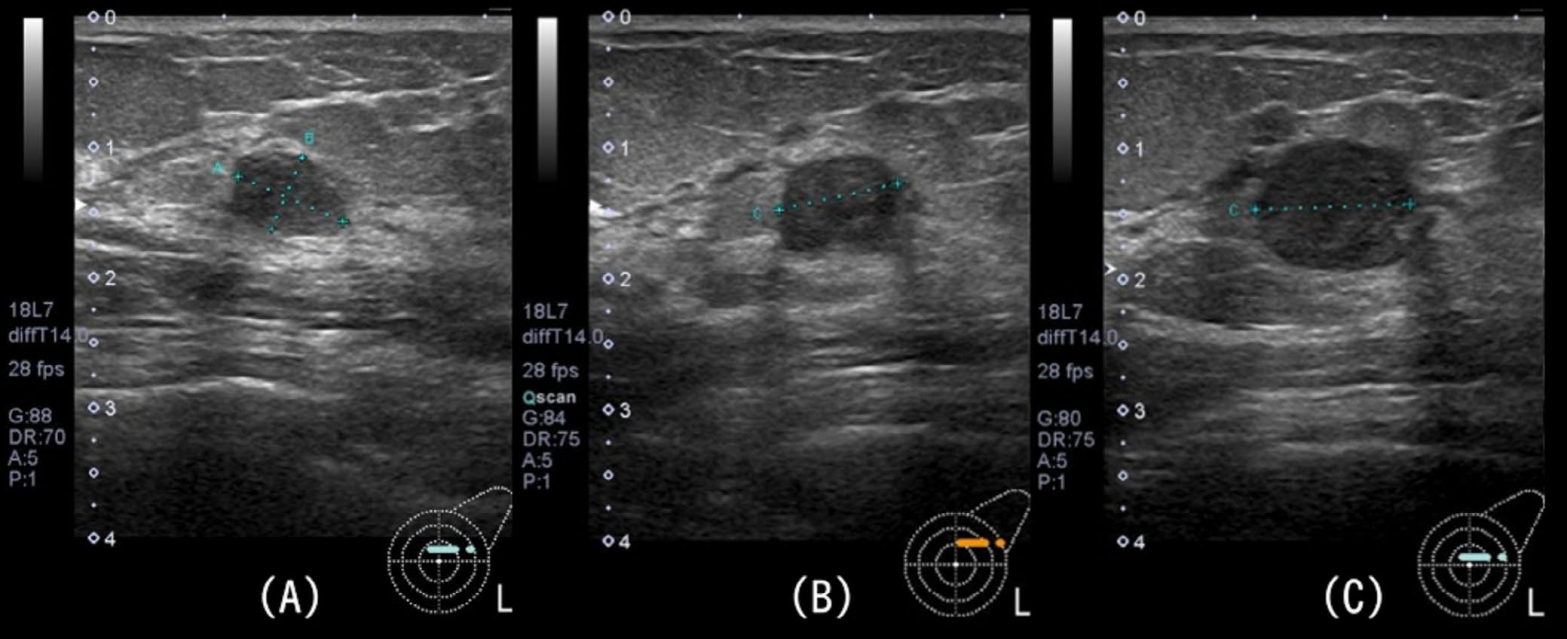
Serial ultrasound images of the left breast mass. (A) Initial presentation: The lesion was identified and diagnosed as an IDP via needle biopsy. (B) Six months later: A slight increase in size was observed. (C) One year later: The lesion demonstrated further enlargement with irregular margins, raising suspicion of malignancy.
